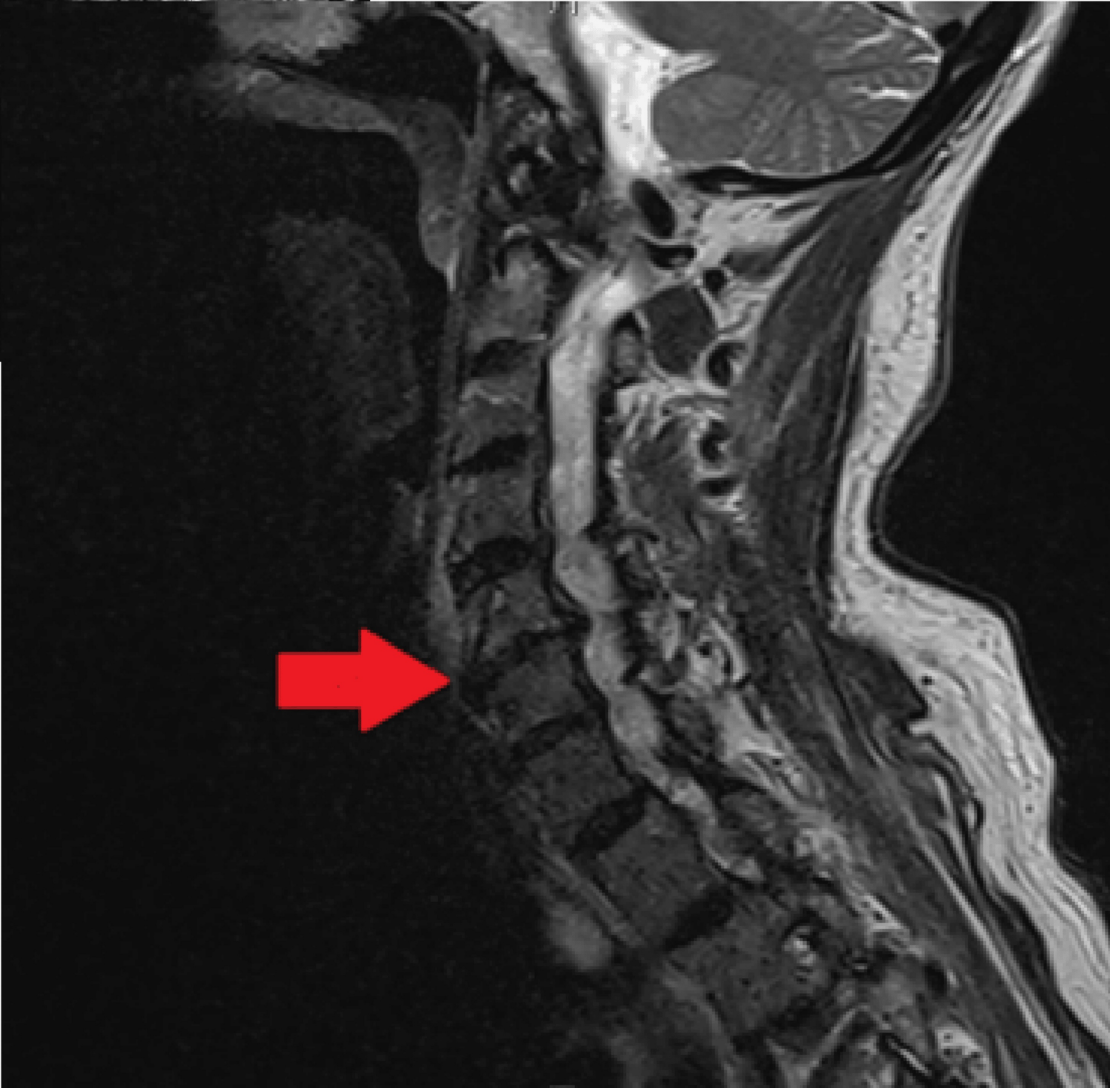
Multiplanar multisequence MRI cervical spine performed without intravenous contrast revealing partial thickness tear of the anterior longitudinal ligament tear, as shown by the arrow
